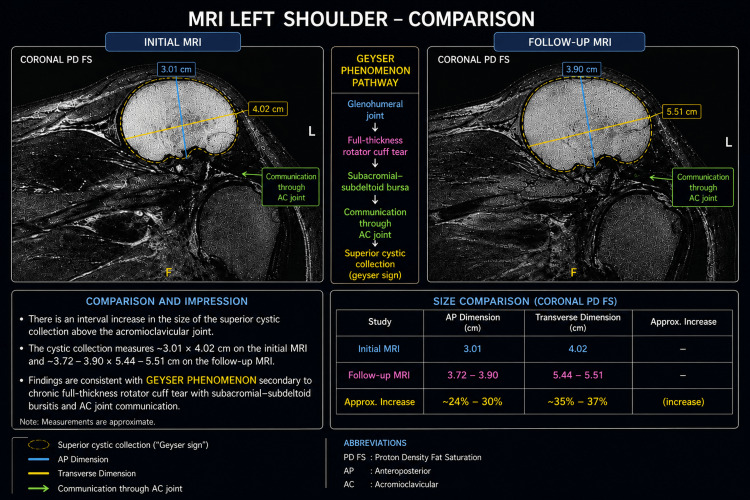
Comparison of the serial MRI of the current patient, demonstrating progressive enlargement of the acromioclavicular (AC) joint cyst (Geyser phenomenon). Initial and follow-up MRI examinations of the patient's left shoulder demonstrated interval enlargement of the cystic lesion, with persistent communication with the acromioclavicular joint. Note: The annotations, labels, and layout were created by the authors using Canva® (Canva Pty Ltd., Sydney, Australia) based on the patient’s original MRI images. No AI-generated images were used.
